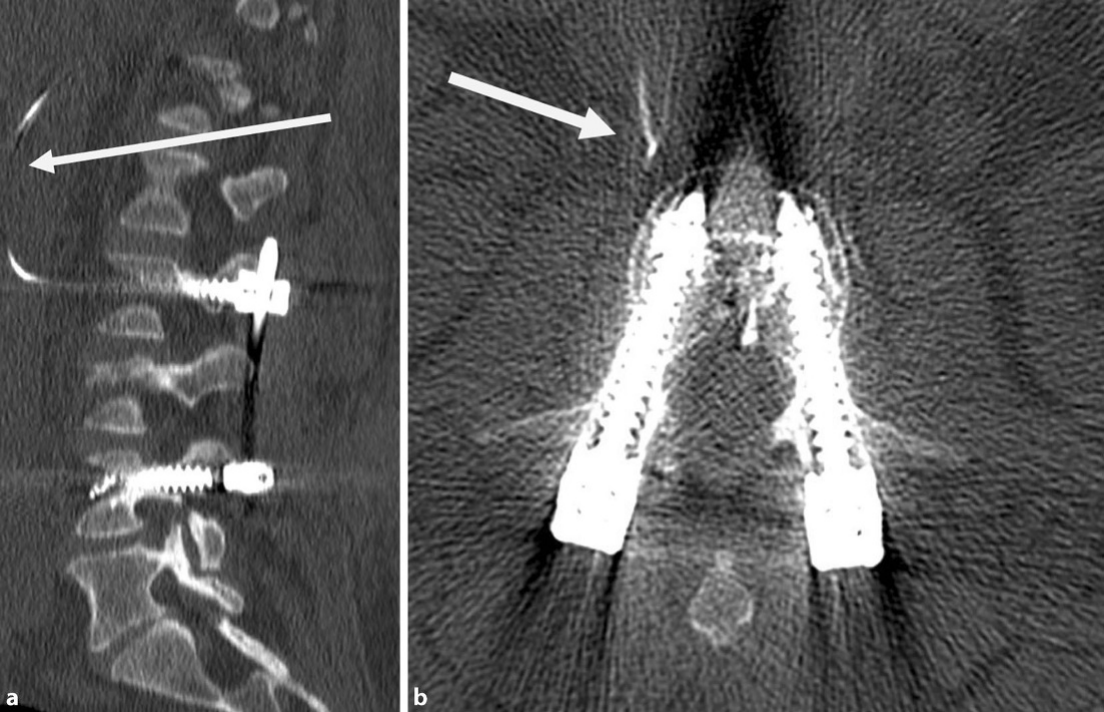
Nach Diskussion des Falls in der Gefäßkonferenz wurde in Abwägung der möglichen Risiken und Notwendigkeit einer Zemententfernung (Wird dadurch eine Embolie provoziert? Wäre eine Zementlungenembolie überhaut symptomatisch? Kann alles Material überhaupt entfernt werden, und wenn ja, wie?) ein Eingriff zur Zemententfernung auch aufgrund der völligen Beschwerdefreiheit der Patientin zurückgestellt. Eine CT-Untersuchung von LWS, Thorax und Abdomen zur Verlaufskontrolle und zum Ausschluss einer Zementembolie wurde empfohlen.
